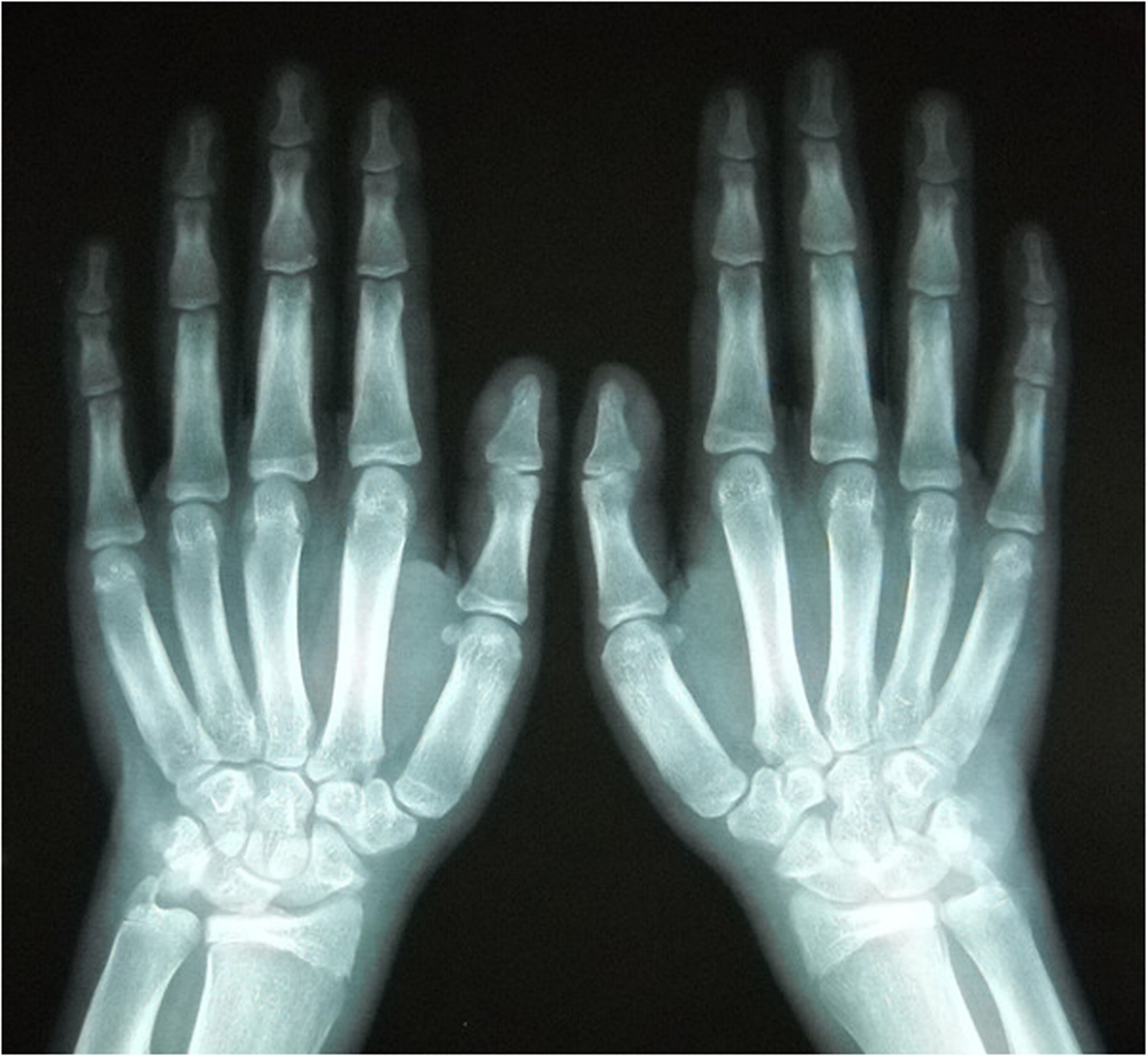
X-ray images of the hands disclosed widening of the epiphysis.
